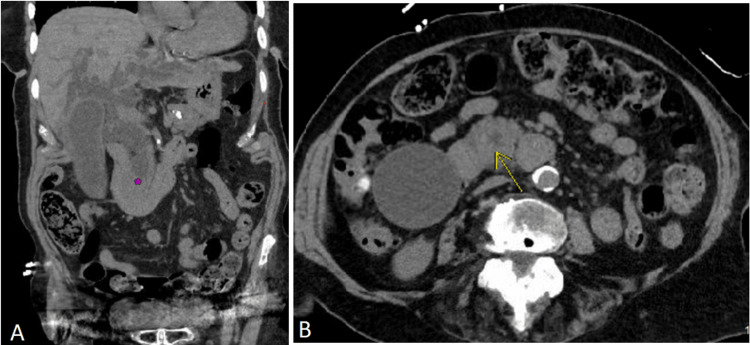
CT scan of abdomen/pelvis demonstrating a mass obstructing the common bile duct. A: Coronal CT image demonstrating a mass (purple asterisk) in the distal-most aspect of the common bile duct with marked intra- and extra-hepatic biliary duct dilatation. B: Axial CT image demonstrating a mass that is partially obstructing the distal common bile duct (yellow arrow).
